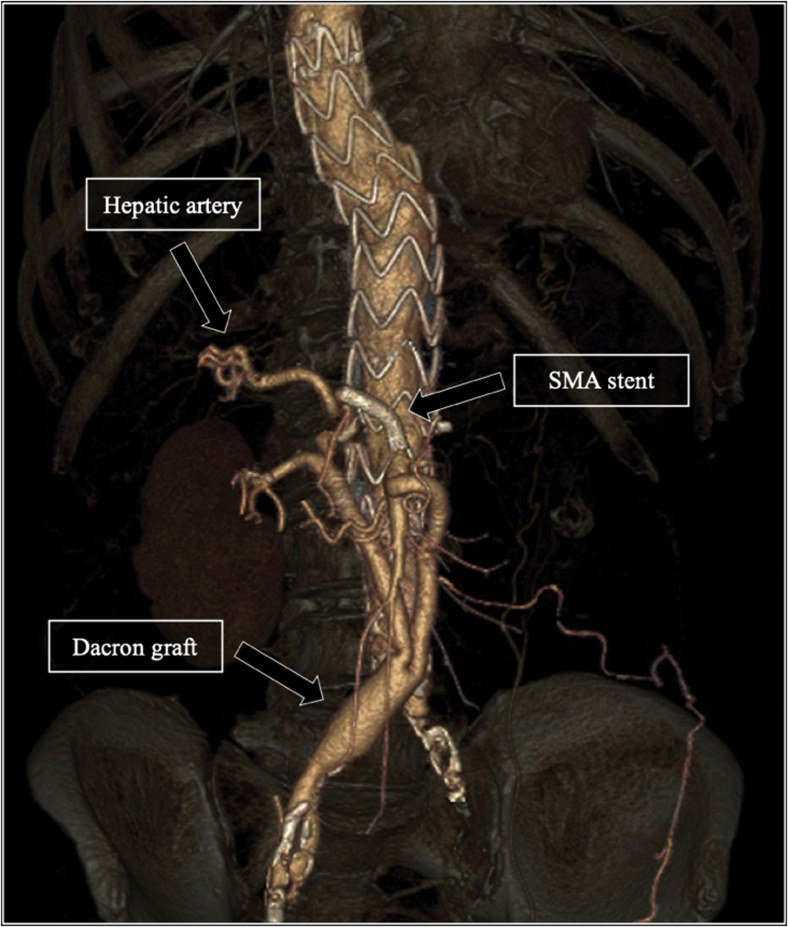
Follow-up computed tomography (CT) angiography of the aorta 12 months after the procedure showing patency of the Dacron graft and the superior mesenteric artery (*SMA*) covered stent.
